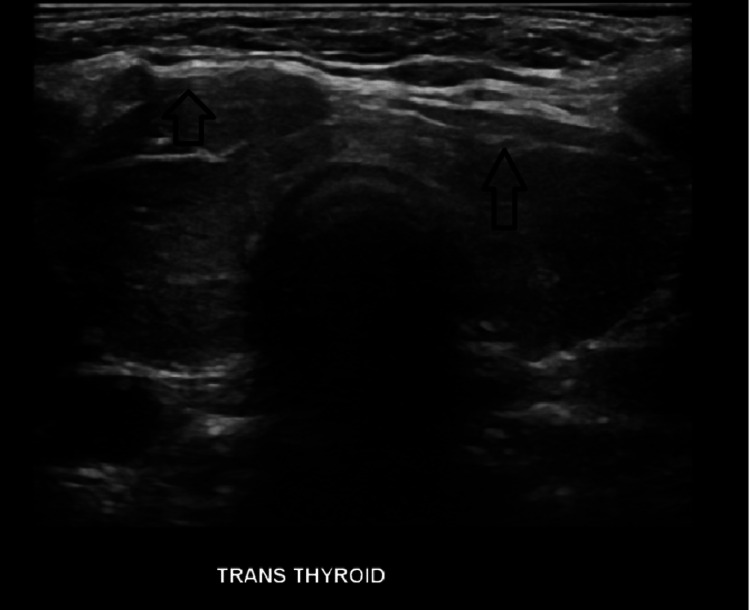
Thyroid ultrasound Thyroid ultrasound showing heterogenous echogenicity of thyroid gland compatible with thyroiditis (arrow).
